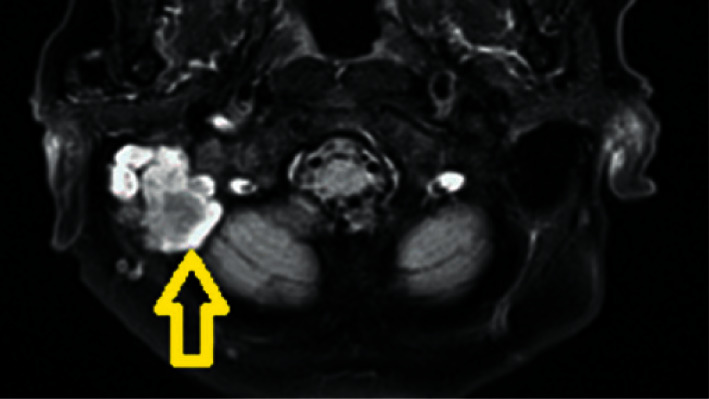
Initial T2-weighted magnetic resonance imaging revealing a mass occupying the right middle ear and mastoid (yellow arrow).
